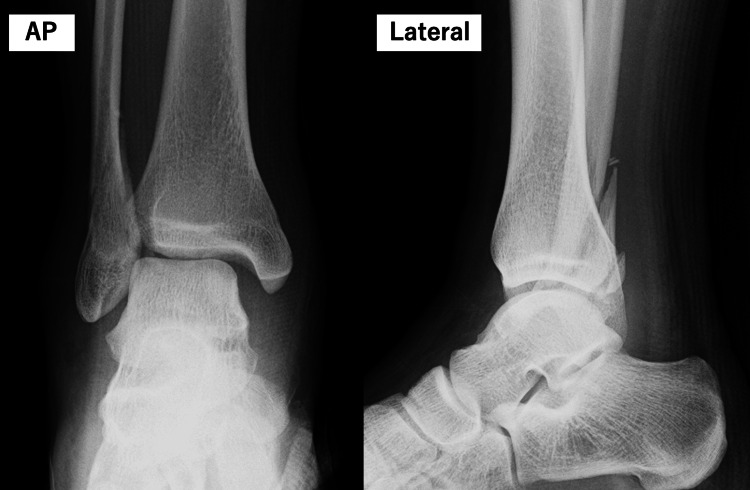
Preoperative X-rays of the right ankle Preoperative anteroposterior and lateral X-ray views of the right ankle demonstrating a SER type stage IV fracture as classified by L-H. L-H, Lauge-Hansen; SER, supination-external rotation
